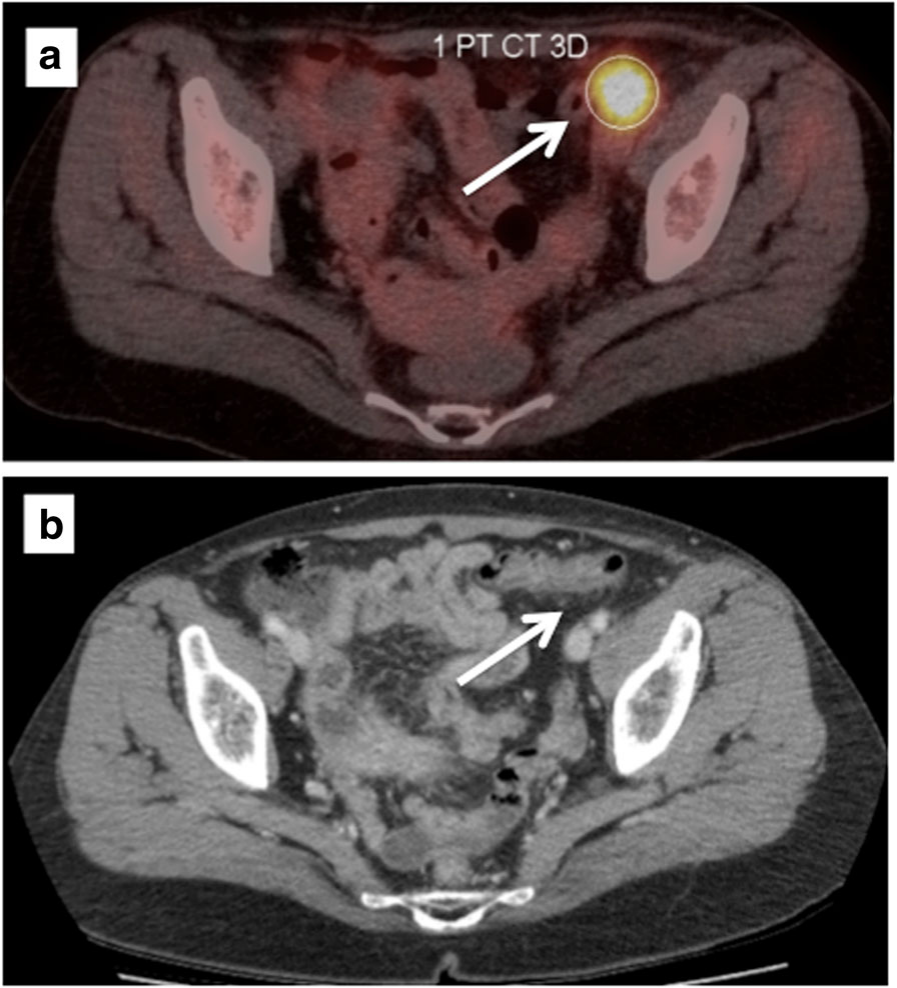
The patient had postoperative complications with infection and lymphatic leakage. Subsequent positron emission tomography-computed tomography (a) showed masses to be growing deeper in the pelvis, which could not be removed surgically (*white arrow*). Computed tomography scanning (b) showed complete remission after four courses of paclitaxel and carboplatin (*white arrow*)
